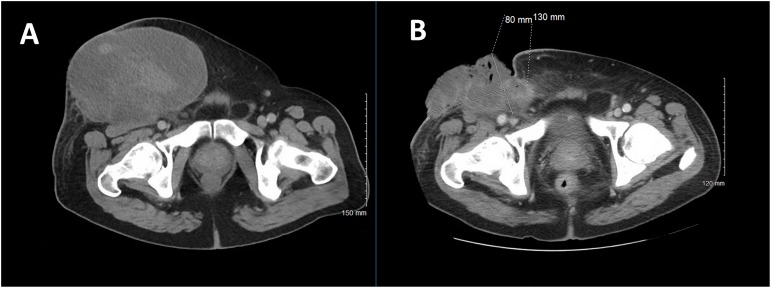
Primary STS at Diagnosis and After Chemotherapy, Plus Radiation A: CT of the abdomen and pelvis reveals a large anterior abdominopelvic mass measuring 19 x 12.8 x 16.8 cm. B: Primary mass after one cycle of chemotherapy (gemcitabine/docetaxel) and local radiation therapy (50 Gy).
